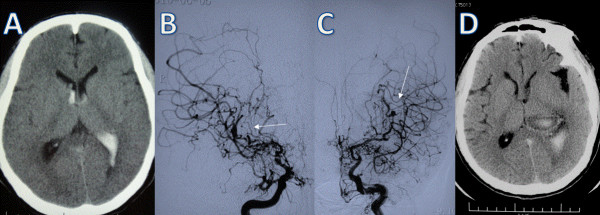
Case 3. (A) Computed tomography scan showing intraventricular hemorrhage involving the left lateral ventricle. (B), (C) DSA demonstrating severe occlusive changes of both intracranial internal carotid arteries. There are two aneurysms (arrows) arising from the distal portion of the bilateral middle cerebral artery. (D) Computed tomography scan showing a recurrent intraventricular hemorrhage.
